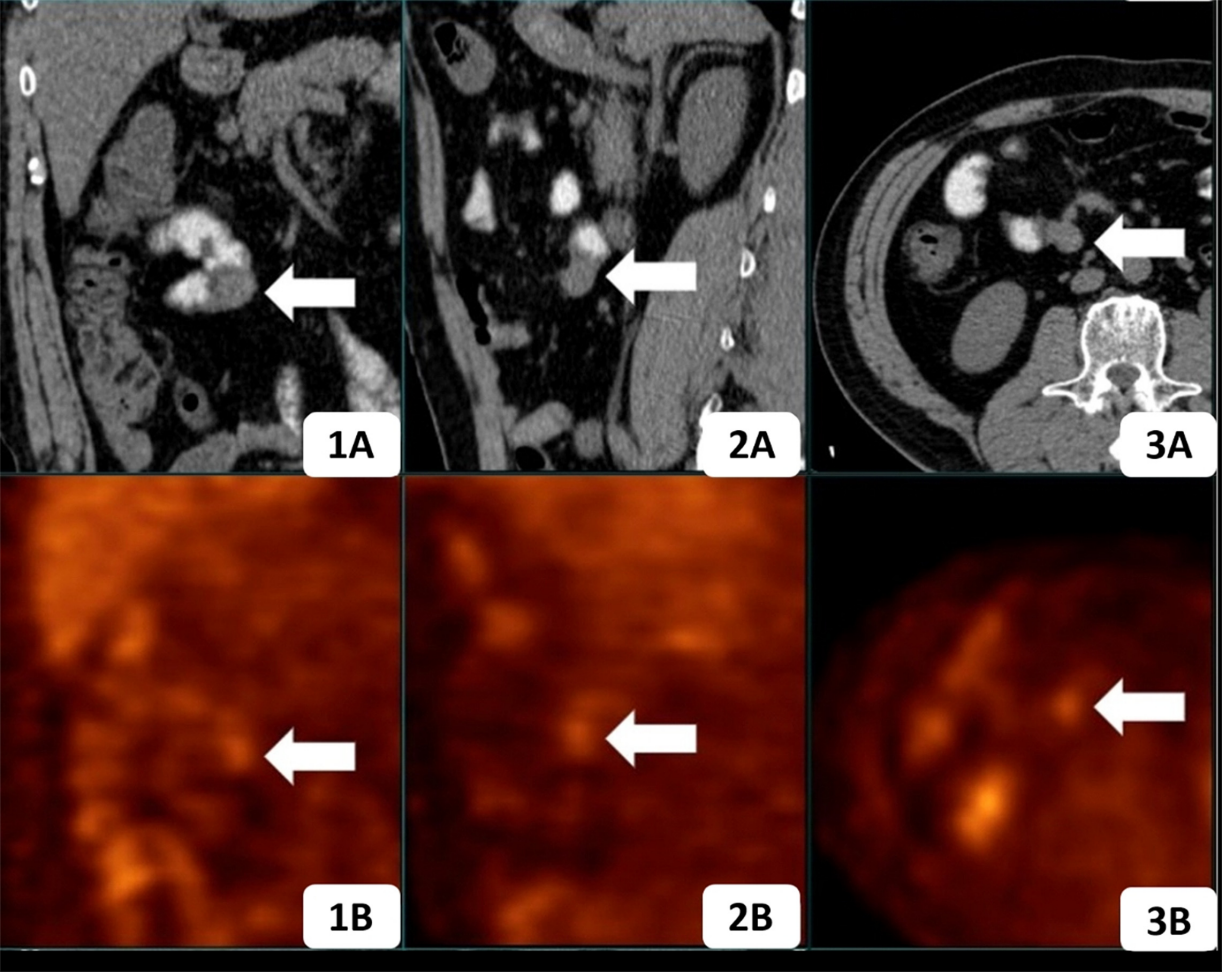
Coronal (1A), sagittal (2A), and axial (3A) abdominal CT with oral contrast showed an obscure small intestinal mass (arrow), with a diameter of 30 mm. The corresponding PET images (1B–3B) revealed slightly accumulated 18F-FDG (arrow), with a maximum standardized uptake value of 2.35.
